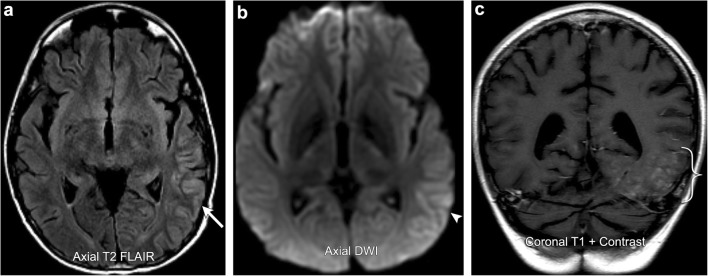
SMART syndrome. A 31-year-old male with a history of posterior fossa atypical teratoid rhabdoid tumour resected and radiated 10 years prior, now presents with persistent headaches, visual field deficits, and 1 day of word-finding difficulties. MRI images demonstrate gyriform thickening and a high T2 FLAIR signal involving much of the posterior cortex of the left temporal, parietal, and occipital lobes (arrow). There is associated mild diffusion restriction (arrowhead) and gyriform enhancement (bracket). Follow-up EEG demonstrated no epileptiform activity and symptoms subsequently resolved. Imaging findings and clinical course compatible with SMART syndrome
